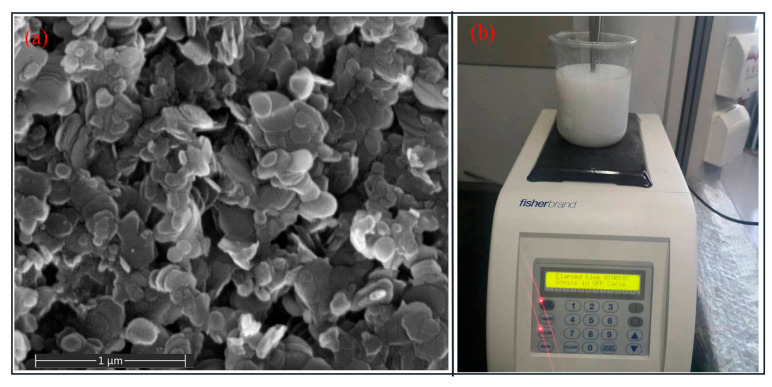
Morphology of hBN (a) and ultrasonic dispersion (b).
